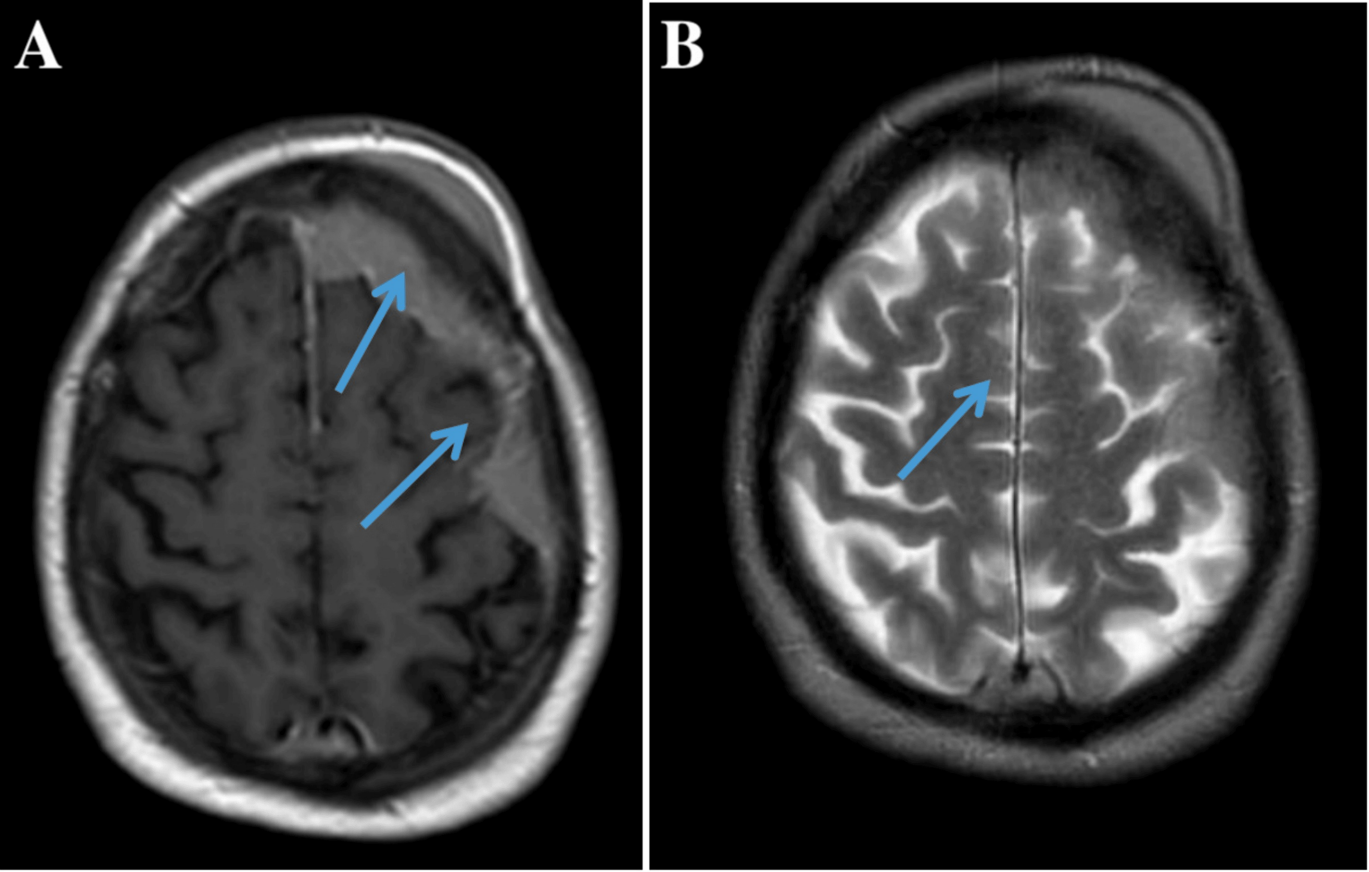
Axial MRI of the right frontoparietal convexity demonstrating an extra-axial dural-based enhancing mass with a dural tail sign (A) T1-weighted post-contrast image showing the homogeneously enhancing mass (arrow) with adjacent dural tail (arrowhead) along the convexity, indicating meningeal involvement. (B) T2-weighted image providing better visualization of the mass's relationship to surrounding cerebrospinal fluid and cortical gyri (arrow), with preserved sulcal spaces suggesting no significant mass effect MRI: magnetic resonance imaging
